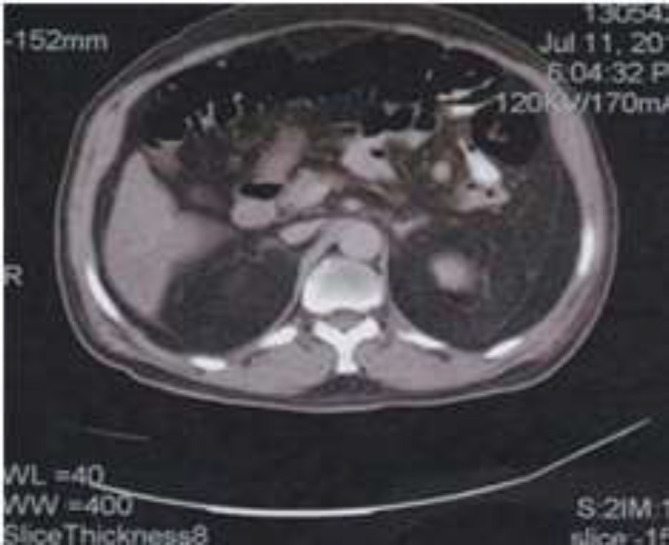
CT scan revealed distended transverse colon
